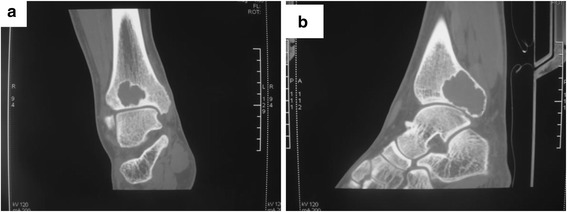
Transection scan of CT shows that the cortex of the distal tibia was destroyed by the tumor; however, fortunately, the medial malleolus was intact, and thus it gives us a chance to keep it. a Anteroposterior CT. b Lateral CT
